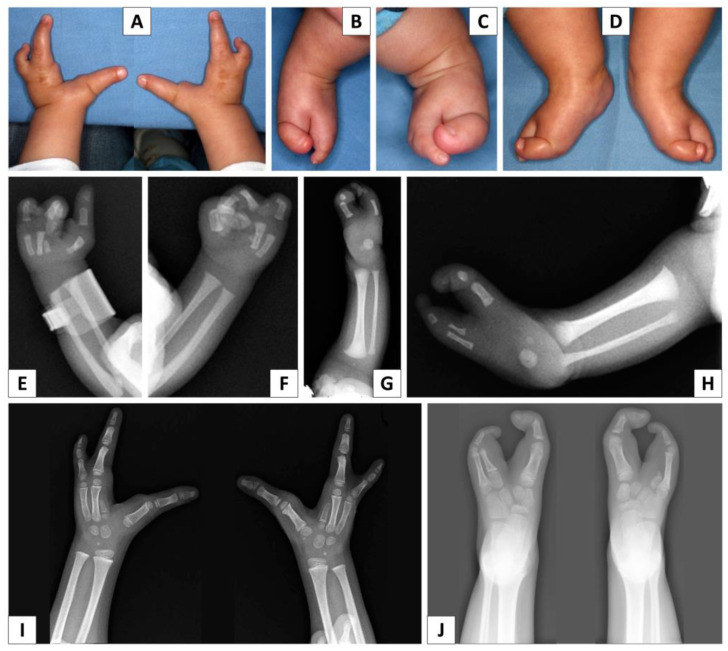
Photographs and X-ray of the hands (A,E,F,I) and feet (B–D,G,H,J) of the proband, showing bilateral SHFM.
